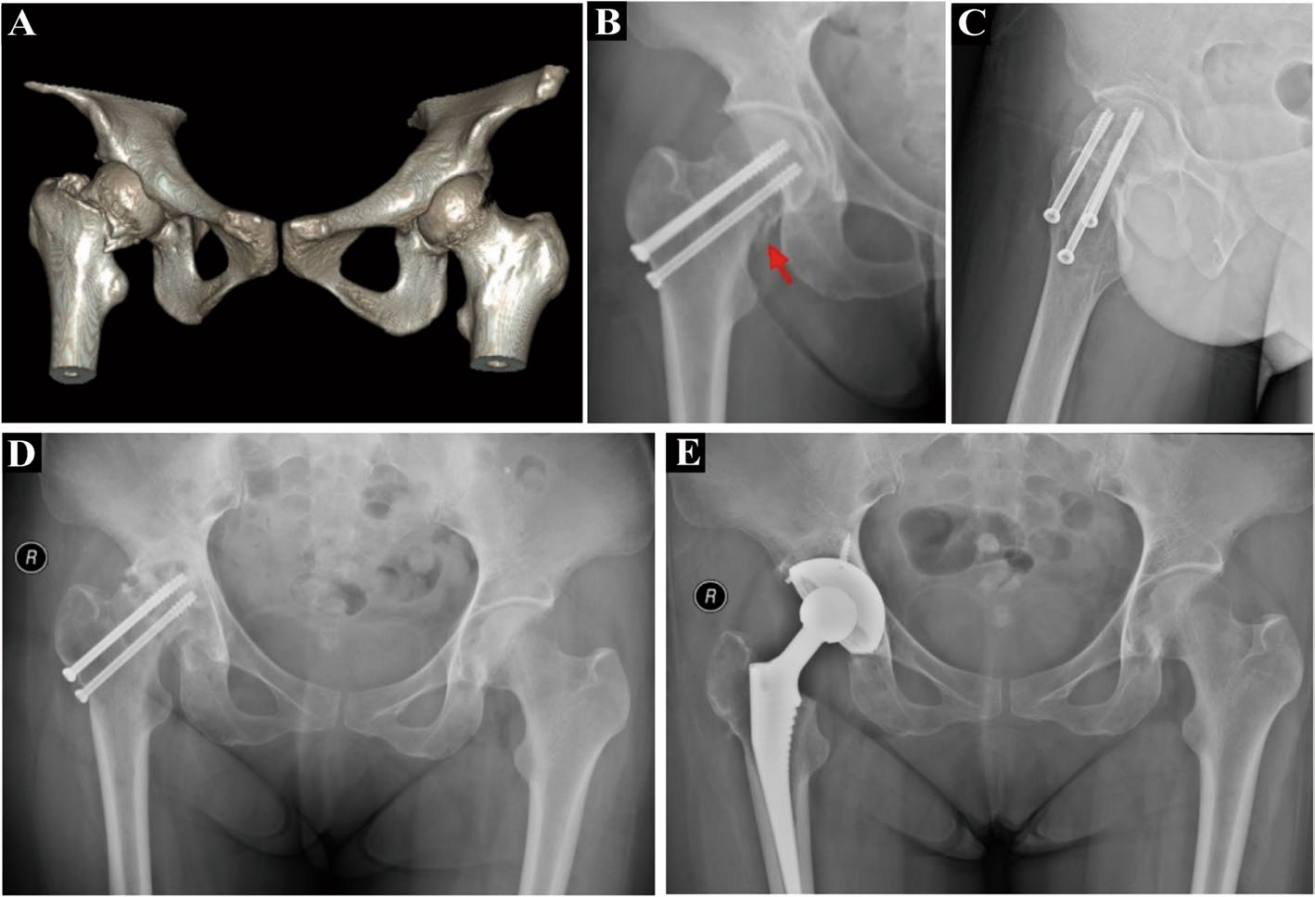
A 48-year-old female patient with femoral neck fracture. A CT scan of femoral neck fracture and double hip; B and C postoperative and lateral X-ray of femoral neck fracture (negative support at the red arrow); D X film 18 months after surgery for femoral neck fracture, femoral head has collapsed and become necrotic; E X-ray after right total hip replacement 18 months after surgery
